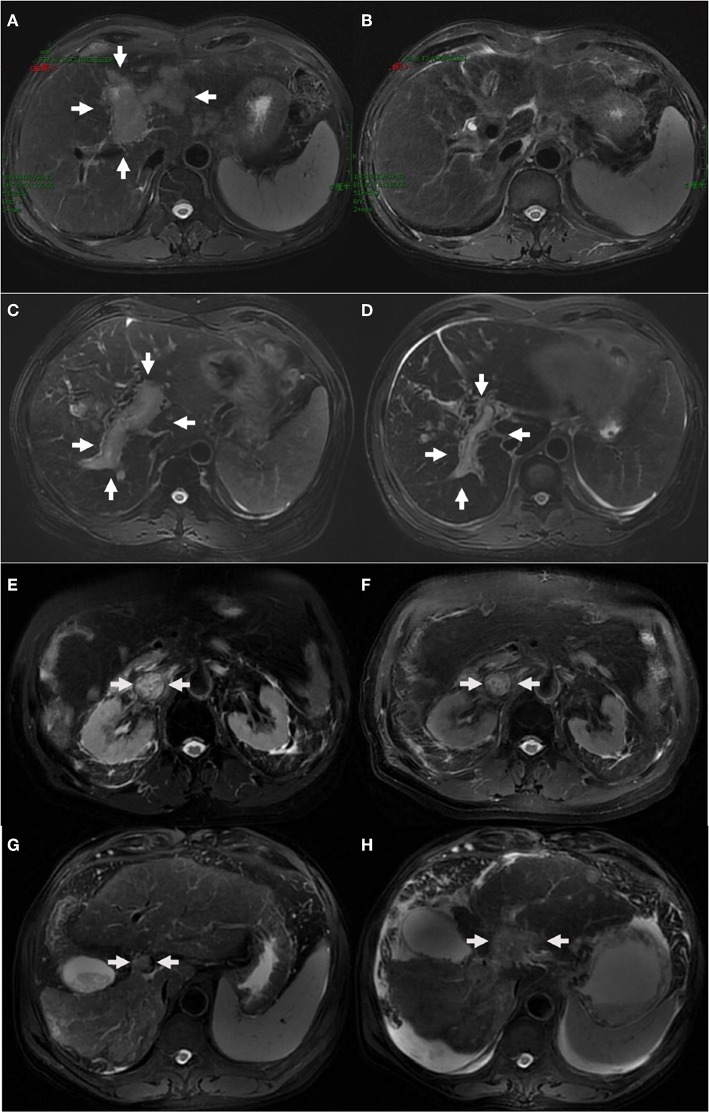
The representative MRI images of HCC with complete response (A,B), partial response (C,D), stable disease (E,F), progressive disease (G,H) before and after treatment. One case with HCC and left portal tumor thrombus (A) obtained complete response 12 weeks after the completion of IMRT, with post-treatment MRI scan showing complete disappearance of MVI (B). One case with HCC and right portal tumor thrombus (C) obtained partial response (D). One case with HCC and inferior vena cava tumor thrombus (E) showed no significant changes after treatment (F). One case with HCC and portal vein tumor thrombus (G) progressed and developed ascites after treatment (H).
